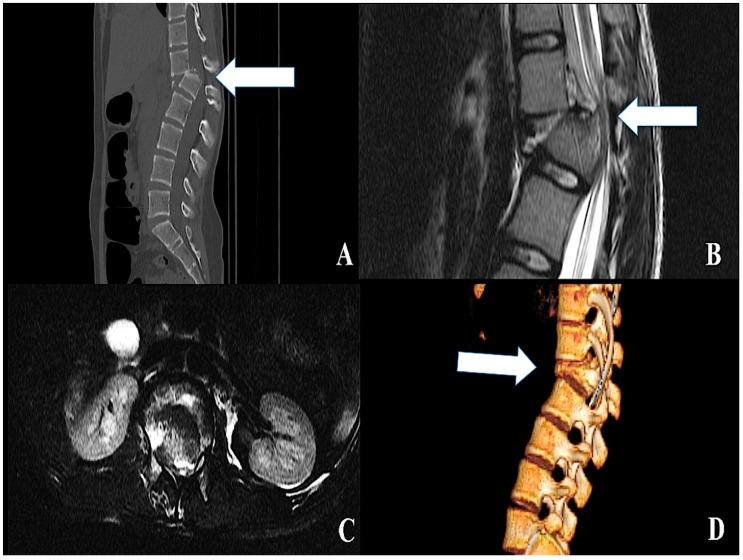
(A) Computed tomography (CT) scan disclosing a Thoracolumbar “C” type Spine Fracture Dislocation (arrow), with (B) and (C) complete Spinal Cord Injury (arrow). (D) 3D reconstruction of the CT scan disclosing the Spine Fracture Dislocation (arrow).
